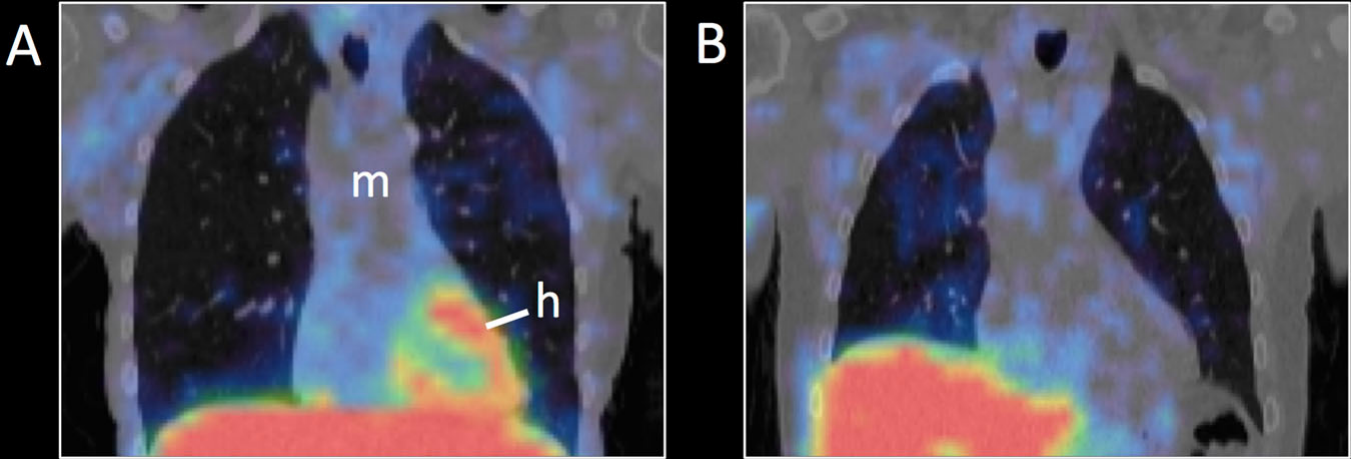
^123^I-MIBG SPECT images superimposed on anatomical CT. a Healthy subject with normal cardiac uptake. b PD patient with severely decreased cardiac uptake. [h heart, m mediastinum]
